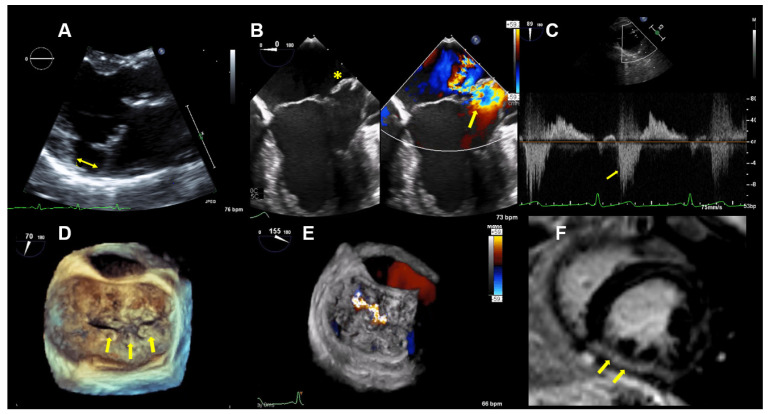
Case 2: Summary of the key findings from multimodality imaging. (A) The mitral annular disjunction (yellow double arrow) is visualized in a 3D transthoracic echocardiography view. (B) A 4-chamber transesophageal echocardiographic (TEE) view shows the bi-leaflet prolapse, which is more pronounced in the posterior leaflet (yellow asterisk), thus resulting in an eccentric mitral regurgitation (MR) jet, as seen in color Doppler (right; yellow arrow). (C) The pulsed-wave Doppler profile derived in the left superior pulmonary vein shows a reverse systolic flow (yellow arrow) as a sign of severe MR. (D) Larger prolapse segments are marked with a yellow arrow in a 3D TEE view. (E) The MR is seen in a 3D TEE enface view with color Doppler. (F) Cardiac magnetic resonance imaging reveals focal mid-wall and subendocardial fibrosis in the mid-inferior wall (yellow arrows).
